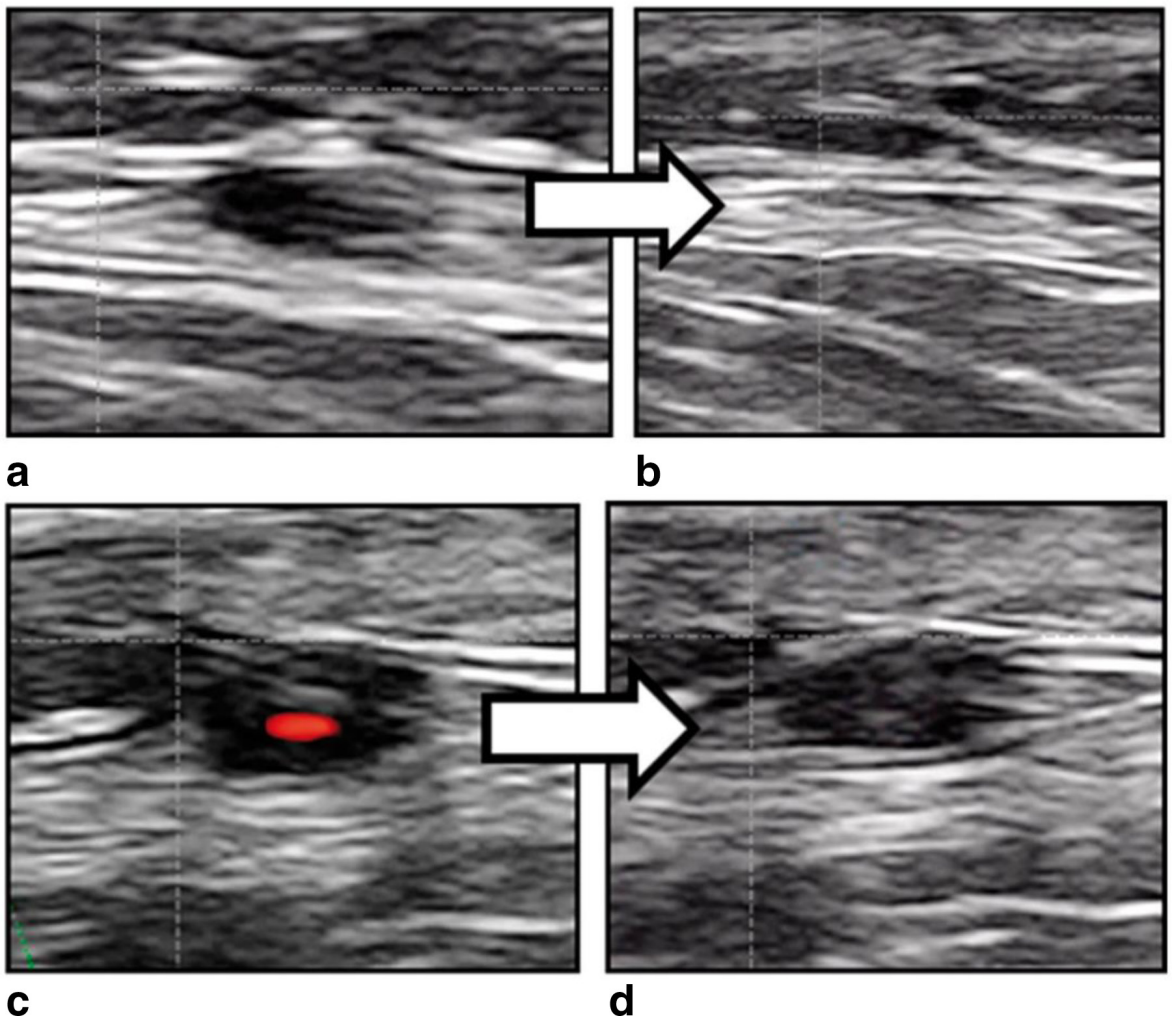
(a) Normal temporal artery prior to compression. (b). Normal temporal artery collapsing under pressure. (c). Inflamed temporal artery prior to compression. (d). Inflamed temporal artery resistant to compression, suggestive of the diagnosis of GCA.Reproduced from Schmidt, AW, Ultrasound in the Diagnosis and Management of Giant Cell Arteritis, Rheumatology, 2018, 57, 2, by permission of Oxford University Press. GCA, giant cell arteritis
